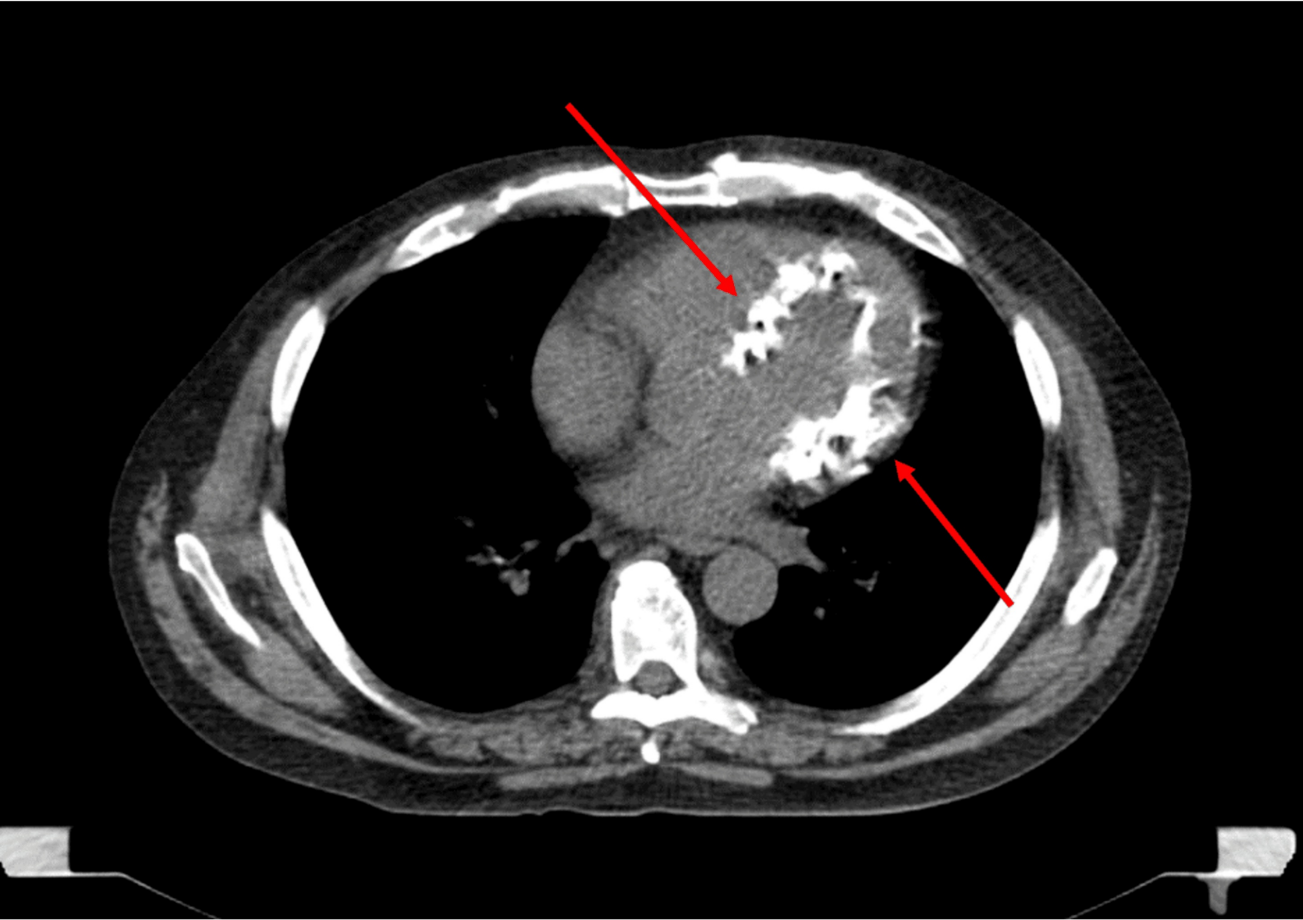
Low-dose CT scan showing bright calcium deposits within the myocardium. Calcium appears as bright as bone CT: Computed tomography
